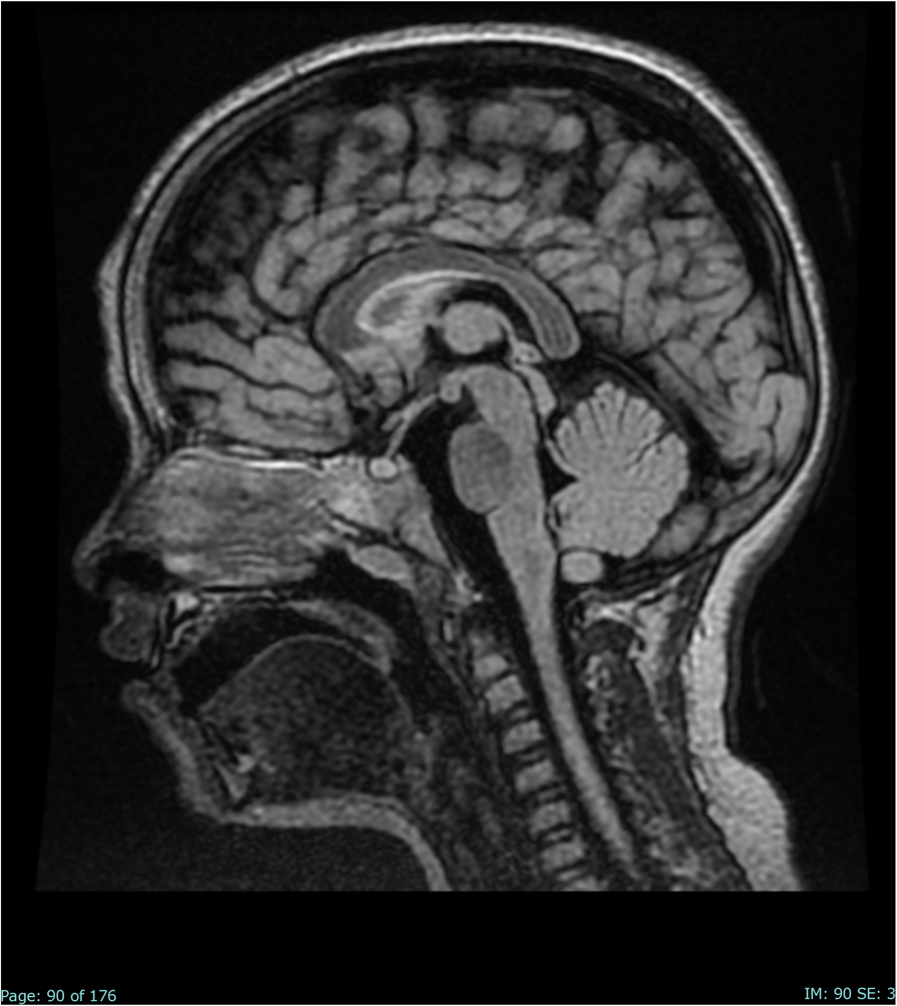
Sagittal FLAIR image on day 40 after admission: the lesion in the splenium of the corpus callosum has completely disappeared
